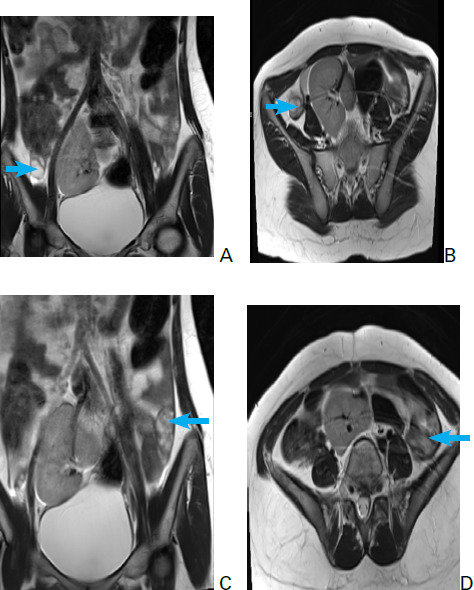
Coronal and axial T2 weighted MR images show the right ovary in more cranial position than normal lateral to the right external iliac artery (A) and (B). Coronal and axial T2 weighted MR images show the left ovary in more cranial position than normal lateral to the left external iliac artery (C) and (D)
